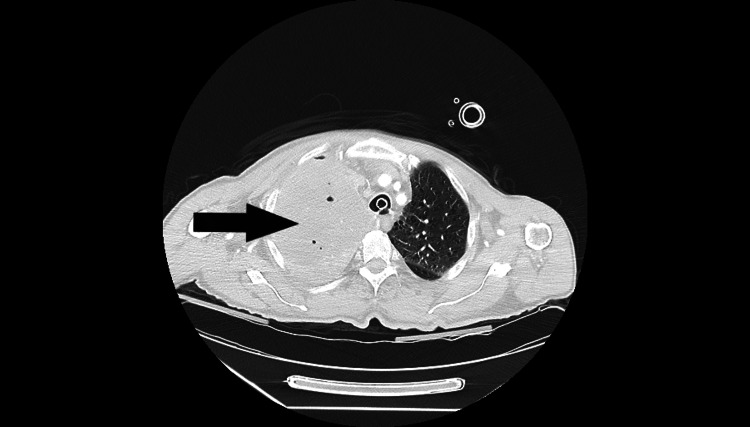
Axial CT chest section. The black arrow showing the right main bronchus obstruction.
